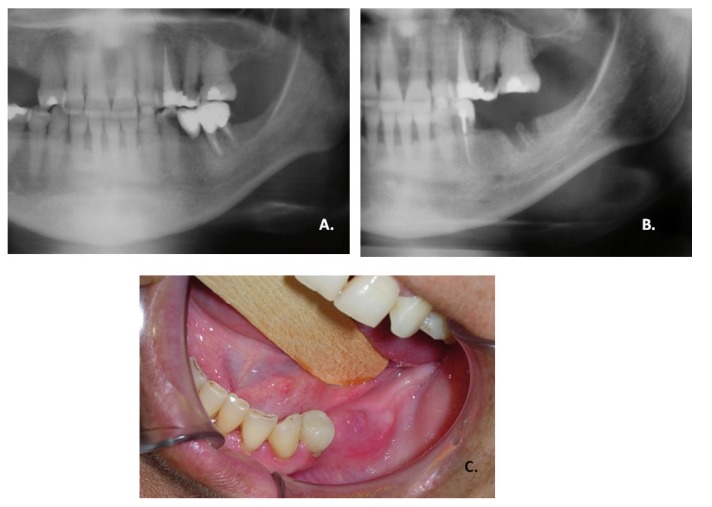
Non-healed socket after exodontia. A. Panoramic radiograph before exodontia. B. Panoramic radiograph after 3 months of exodontia; image suggests bone remodeling did not occurred in alveolar socket. C. Clinical aspect of alveolar socket after exodontia without mucosa coverage.
